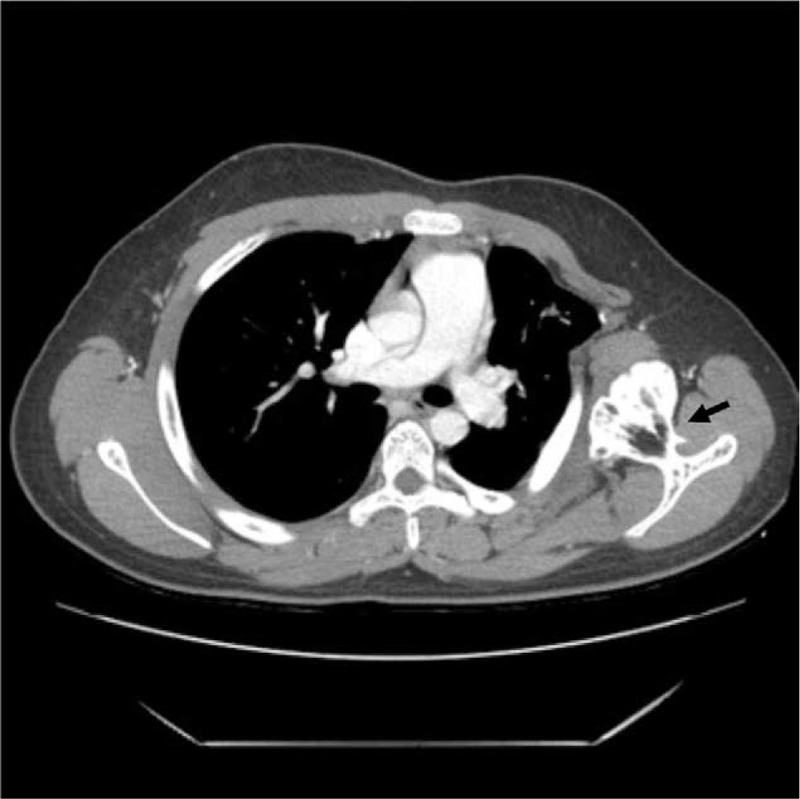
In chest CT, about 5.0 × 4.6 × 5.7 cm sized pedunculated bony lesion is noted in the ventral aspect of left scapula, which shows the continuity of bony cortex and medulla.
